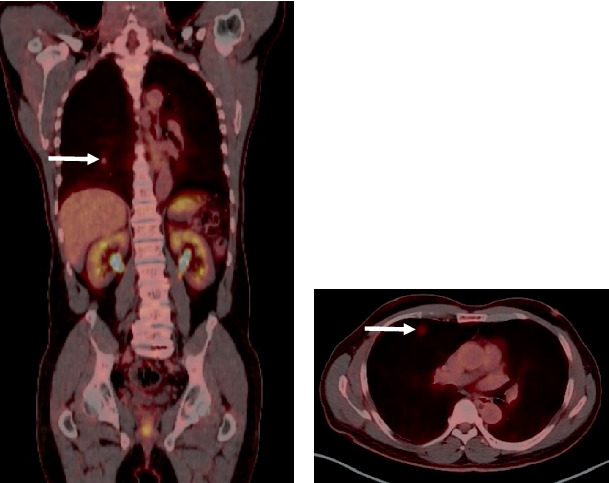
FDG positron emission tomography (PET) scan showing a 1-cm right upper lobe pulmonary nodule with mild FDG avidity (SUV 2.1) in both (a) coronal view and (b) axial view.
